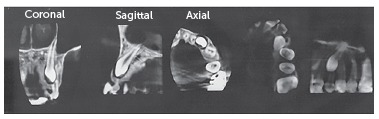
Pretreatment CBCT image.
